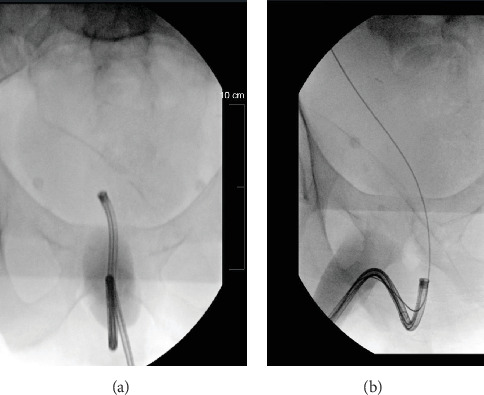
(a) Anterior to posterior and (b) lateral x-ray views of flexible cystoscope course to the bladder including safety wire tortuosity with wire advanced into the right upper tract.
